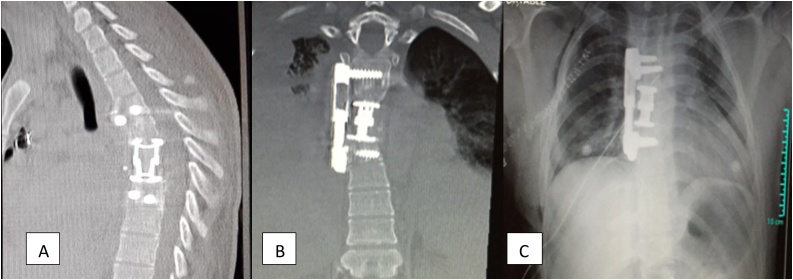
Post-operative CT scan coronal image (A & B) and chest X-ray image (C) showing fusion with cage and screw plate fixation.
